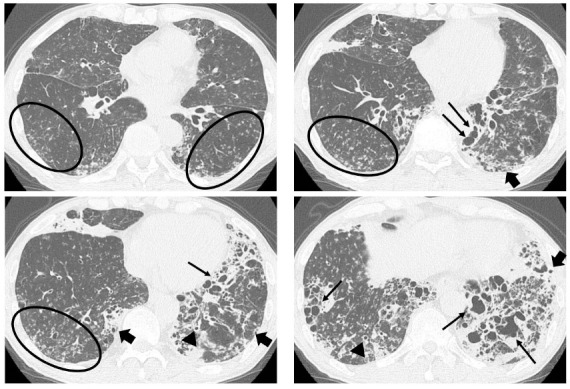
HRCT in a case of severe bronchiectasis. Legend: Bronchial dilatation (thin arrow), visibility of peripheral airway (thick arrow), bronchial wall thickening (triangle), and small airway abnormality (circle) were found in both lungs.
